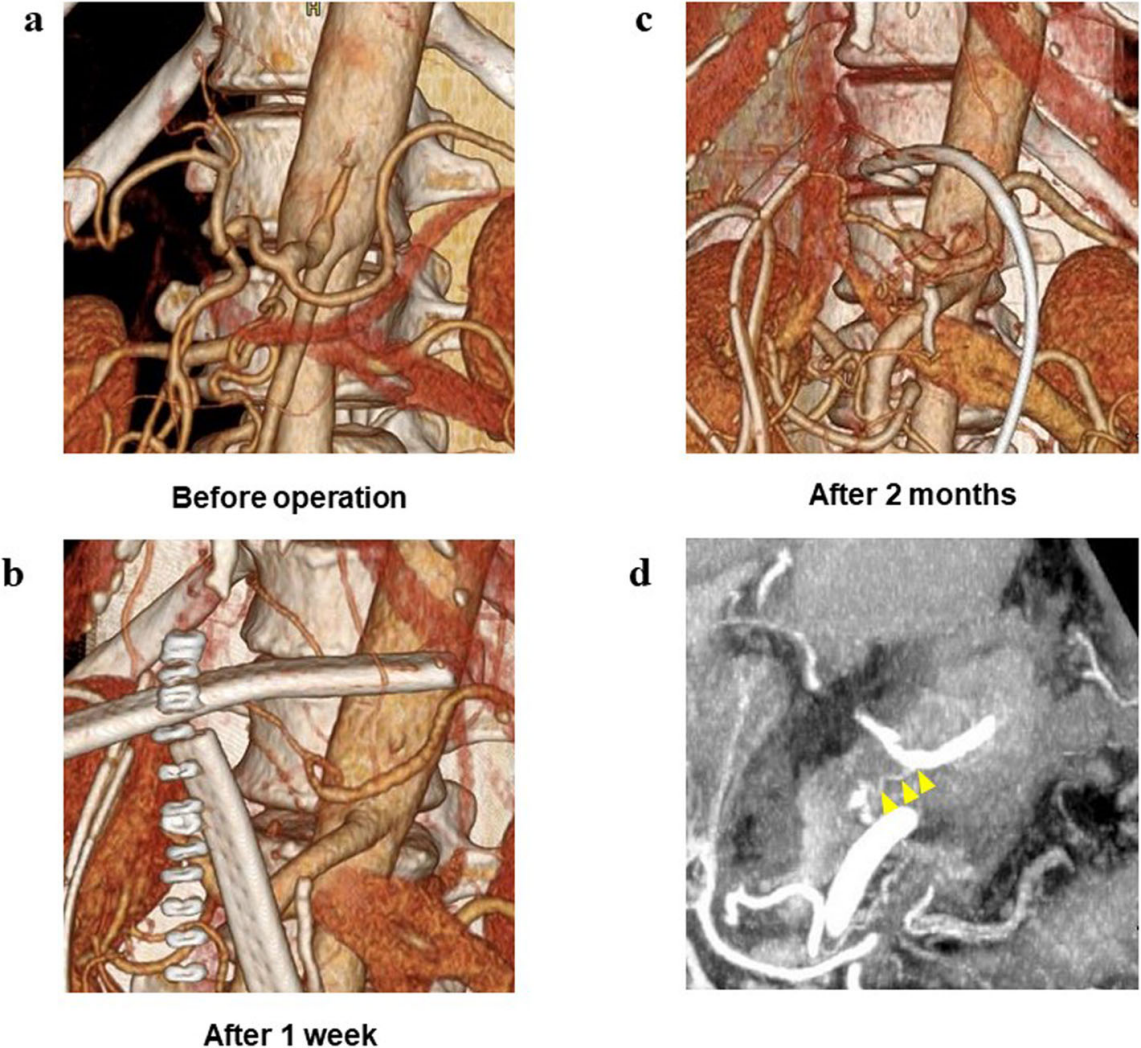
Time course of 3D CT angiography. a Preoperative CT; stenosis of the root of celiac artery. b CT at 1 week after the operation; absence of the root of celiac artery and the reduction of liver blood flow. c CT at 2 months after the operation; collateral circulation from dorsal pancreatic artery with recovery of liver blood flow. d Dorsal pancreatic artery flowed into splenic artery (1 week after the operation)
